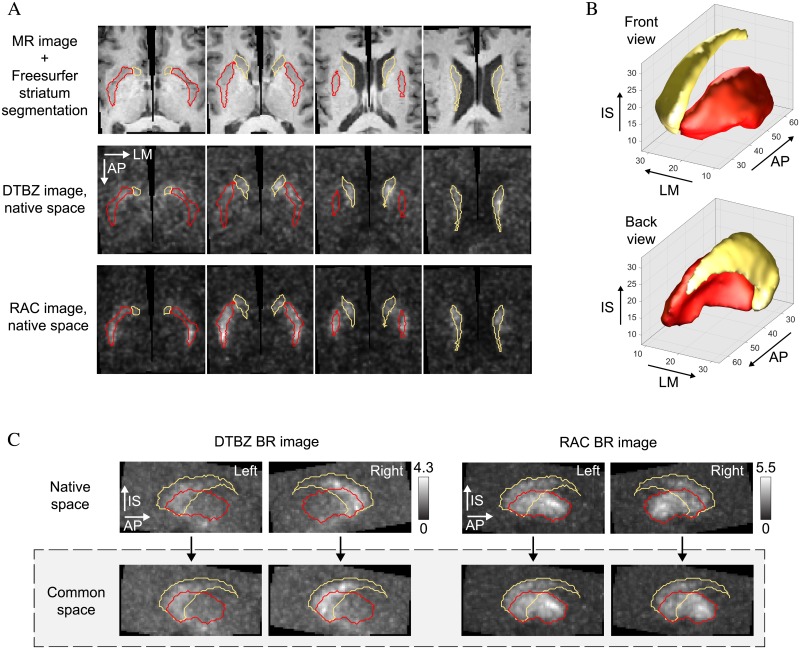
Examples of striatum images and segmentations in subject-native and common spaces. A: Segmented MR image, DTBZ and RAC images in the native space for a PD subject. B: Rendering of the striatal template from two views. To generate the template, Freesurfer segmentations of the MR images of the putamen and caudate in the HC subjects were rigidly co-registered and averaged (N = 10, one side chosen randomly from each subject). C: Comparison of DTBZ and RAC BR images in the subject-native and common spaces (PD subject, same as A). AP = anteroposterior, LM = lateromedial, IS = inferosuperior.
